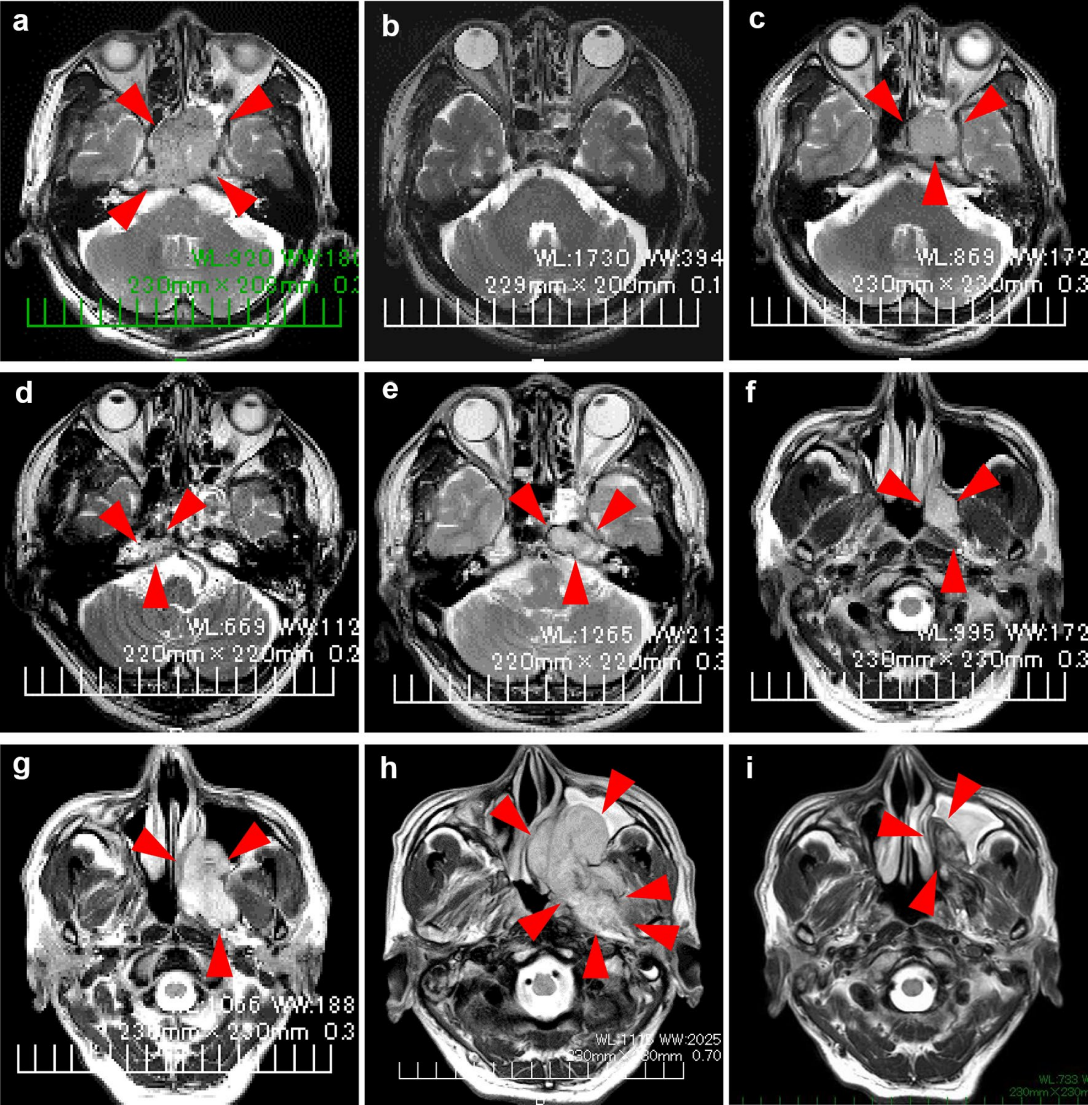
T2-weighted magnetic resonance images of the tumor at different stages of its development. a preoperatively; b 1 month postoperatively; c at first recurrence, 4 months postoperatively; d at second recurrence, 3 months after irradiation. The tumor has recurred outside the irradiated area; e 9 months after the first irradiation. The tumor has extended into the left cavernous sinus; f the tumor extends toward the maxillary sinus 16 months after initial irradiation; g 18 months after the first irradiation, the tumor continues to grow; h after two doses of an immune checkpoint inhibitor (pembrolizumab), the tumor is still growing; and i after the third dose of pembrolizumab, the tumor begins to shrink. It continues to shrink for the remainder of the course of treatment (seven doses in total)
